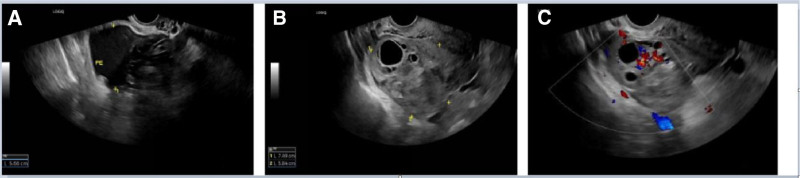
Transvaginal ultrasound characteristics for the second-time examination. (A) Ultrasound images showed that the depth of the pelvic effusion increased to 5.7 cm after 2 hours. (B) A 7.4 cm × 5.8 cm mixed-echo mass with indistinct margins was noted adjacent to the right ovary, with increased pelvic fluid (5.7 cm). (C) Color Doppler image showed that there were blood flow signals within the mass.
